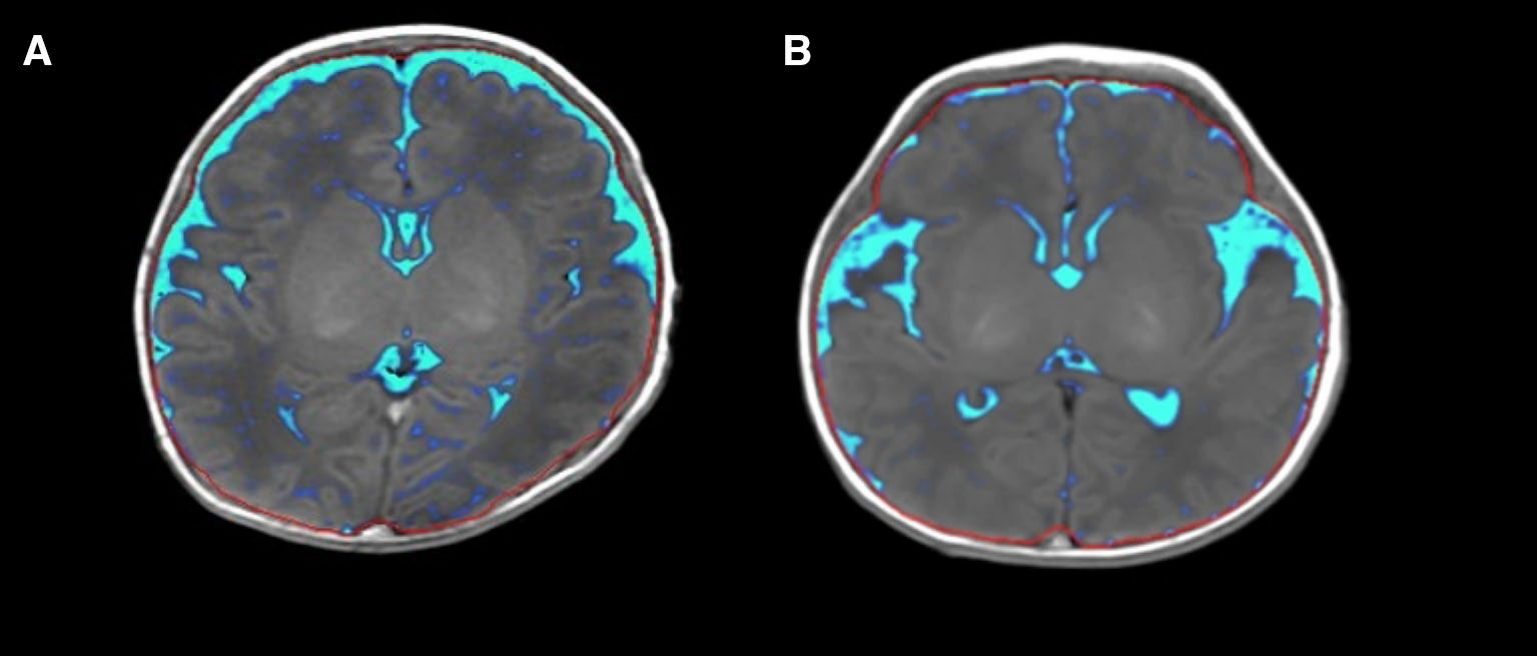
The volume of CSF in preterm infant brain with low-grade IVH (A) and without low-grade IVH (B). The two cases were all male, 38 weeks. Brain volume segmentation by Synthetic MRI. Cerebrospinal fluid, CSF.
